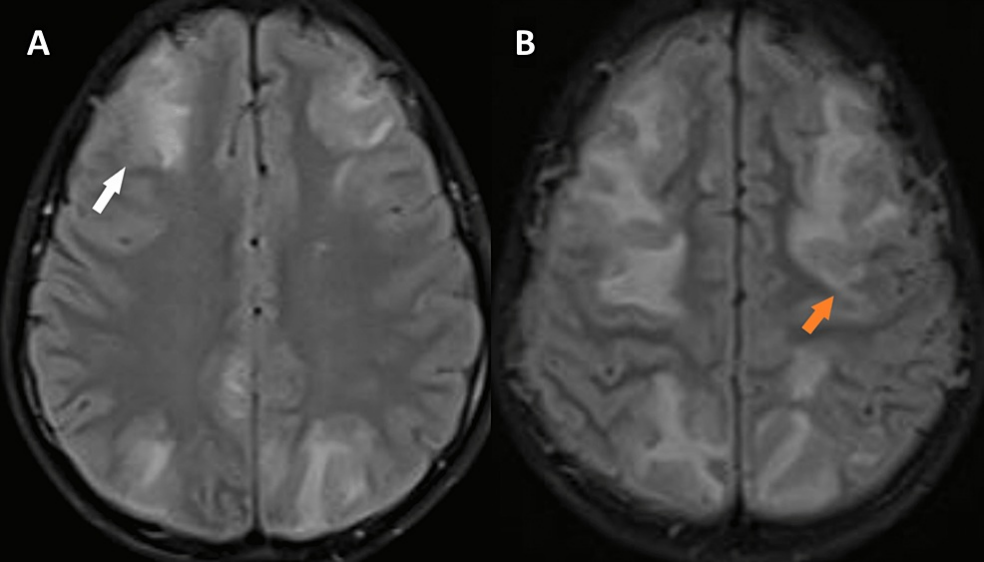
Brain magnetic resonance imaging FLAIR sequence at presentation Brain MRI FLAIR sequence showing symmetrical hyperintensities in both fronto-parietal-occipital cortex and subcortical region involving superficial (A-white arrow) and deep watershed regions (B-orange arrow). FLAIR: fluid-attenuated inversion recovery
